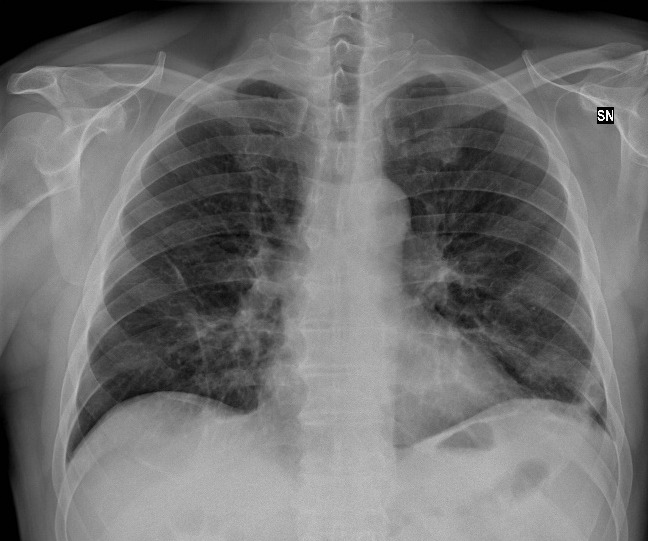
Radiologist declared radiograph negative (same patient as Figure 6)
